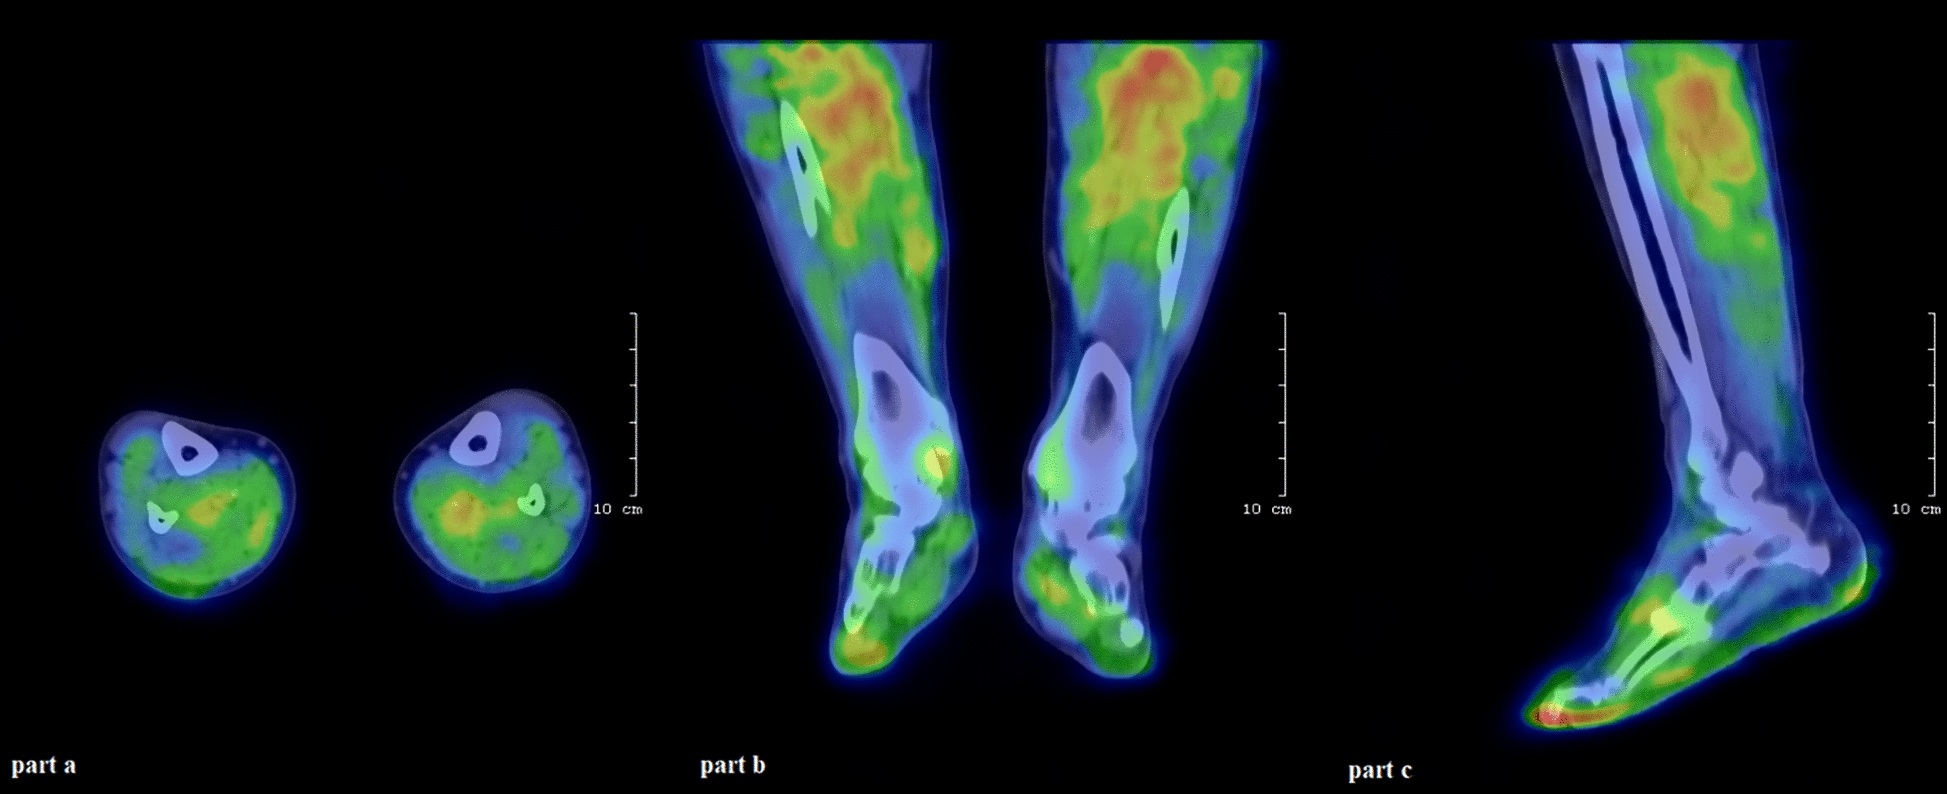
Fused transaxial (a), coronal (b), sagittal (c) [99mTc]Tc-HMPAO SPECT/CT images of the lower extremities obtained 15 min post tracer injection with AnyScan^®^ TRIO SPECT/CT device (Mediso Ltd., Budapest, Hungary). The colours indicate the degree of the radiopharmaceutical uptake of the lower limbs that well correlates with perfusion. (The brighter the color (red) the better perfused the region is). *HMPAO* hexamethylpropylene amine oxime, *SPECT/CT* single-photon emission computed tomography/computed tomography
